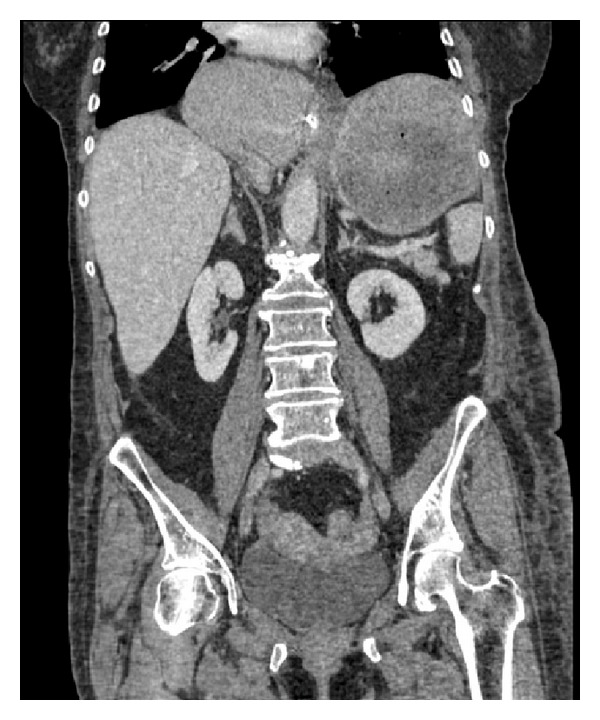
Coronal CT showing herniated stomach above the diaphragm (arrow).
